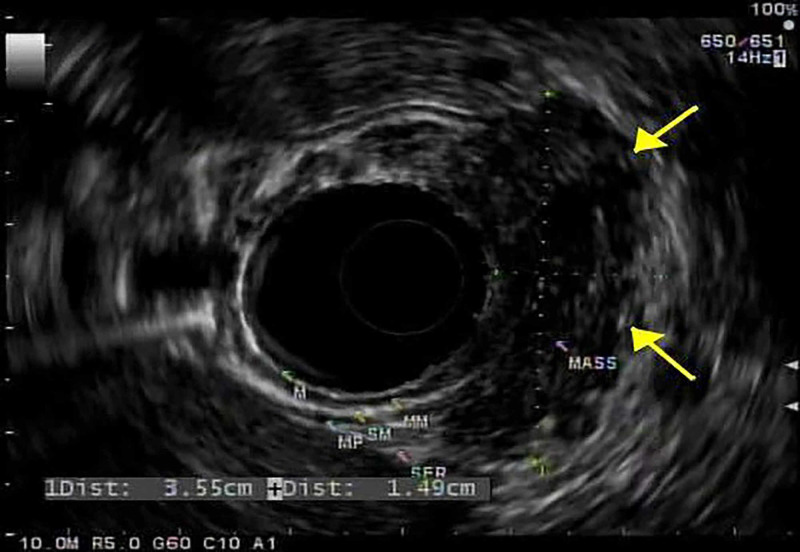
EUS showing a hypoechoic mass in the stomach incisura angularis (yellow arrows) EUS: endoscopic ultrasonography.
